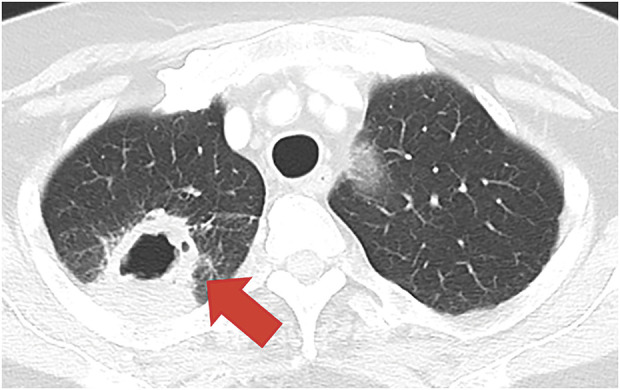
Chest computed tomography 5 months after drug discontinuation. The mass regrew to 21 mm, accompanied by cavity formation and wall thickening (red arrow).
